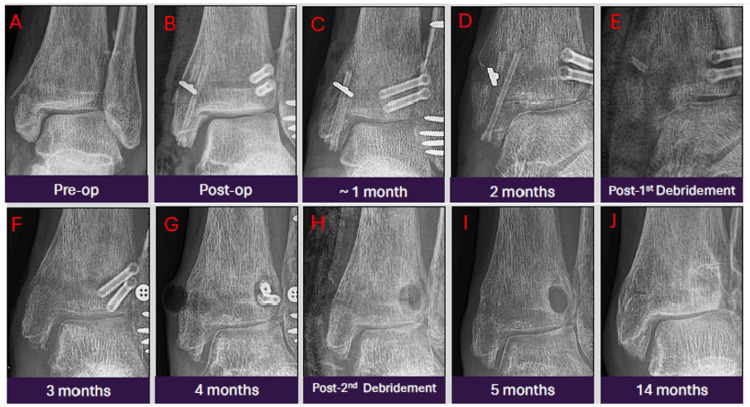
Serial antero-posterior radiographic images from pre-operation to 14 months post-operation showing pathological changes, such as screw extrusion, breakage and infective changes. The radiographs were taken at pre-operation (A), immediately post-operation (B), one month post-operation (C), two months post-operation (D), post-first debridement (E), three months post-operation (F), four months post-operation (G), post-second debridement (H), five months post-operation (I), and 14 months post-operation (J).
